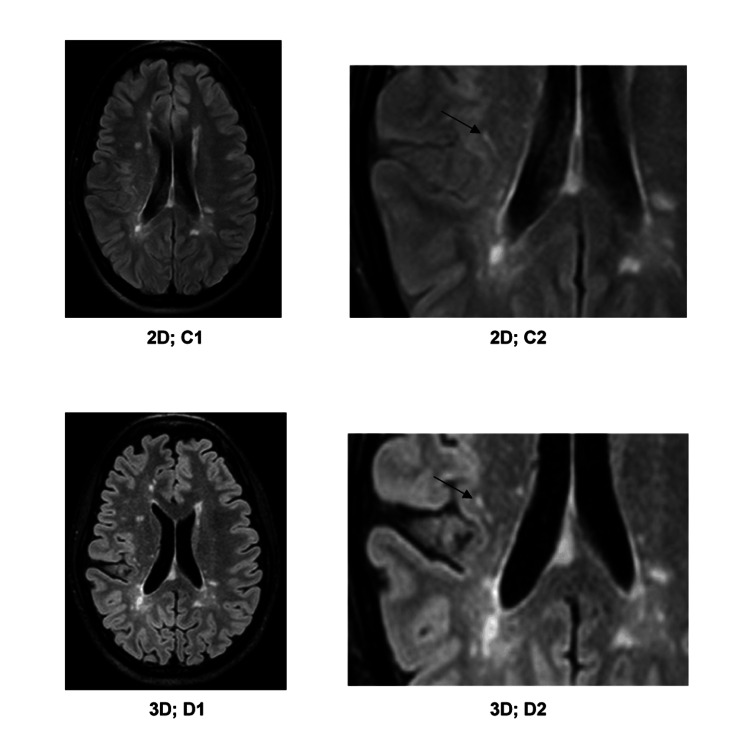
C1) 2D-FLAIR image; C2) detailed enlargement of C1; D1) 3D-FLAIR image, roughly aligning with C1; D2) detailed enlargement of D1. The right parietal lesion (marked with an arrow in C2) actually consists of two separate lesions (marked with an arrow in D2). White matter lesions exhibit clearer differentiation on the 3D-FLAIR image.
